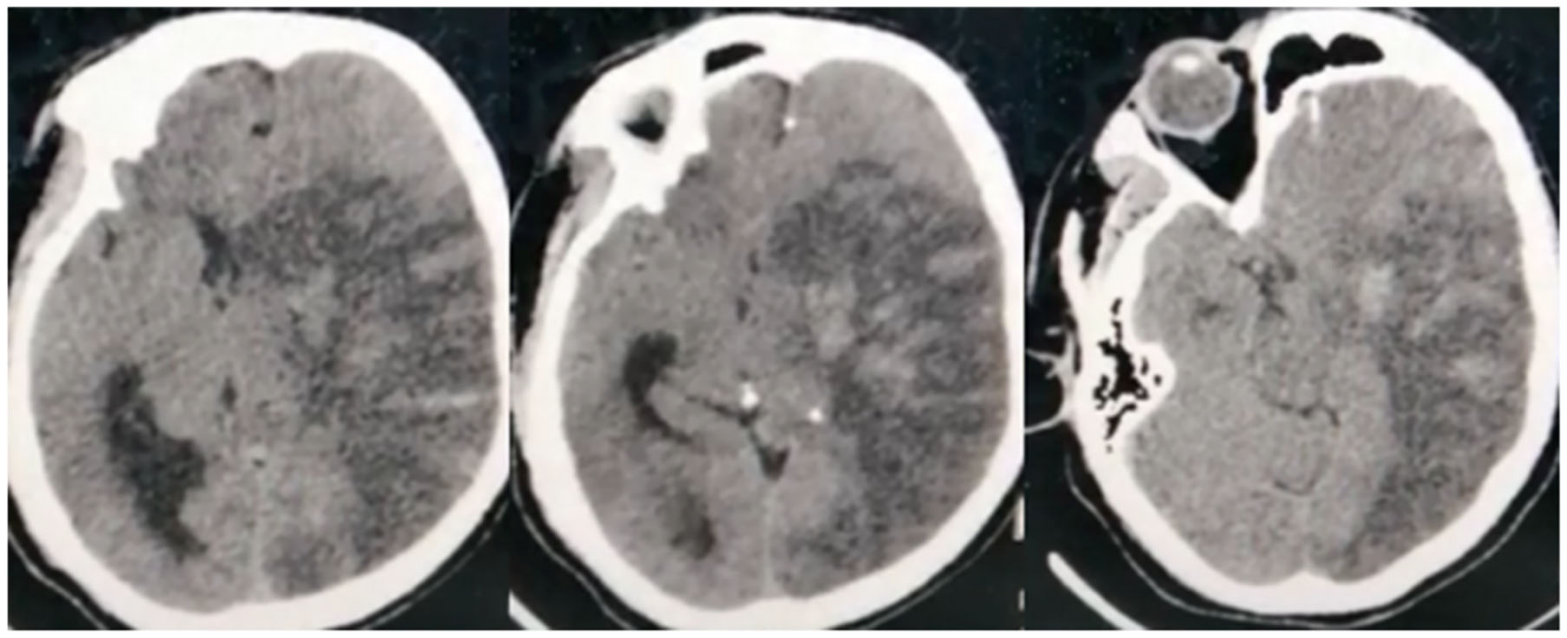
Non-contrast CT brain of a 45-year-old male shows subacute left middle cerebral artery (MCA) territory infarct (large vessel occlusion), associated with marked edema excreting mass effect on the lateral ventricle, and midline shift. Noted multiple hyperdense patches within the infarct representing hemorrhagic transformation.
